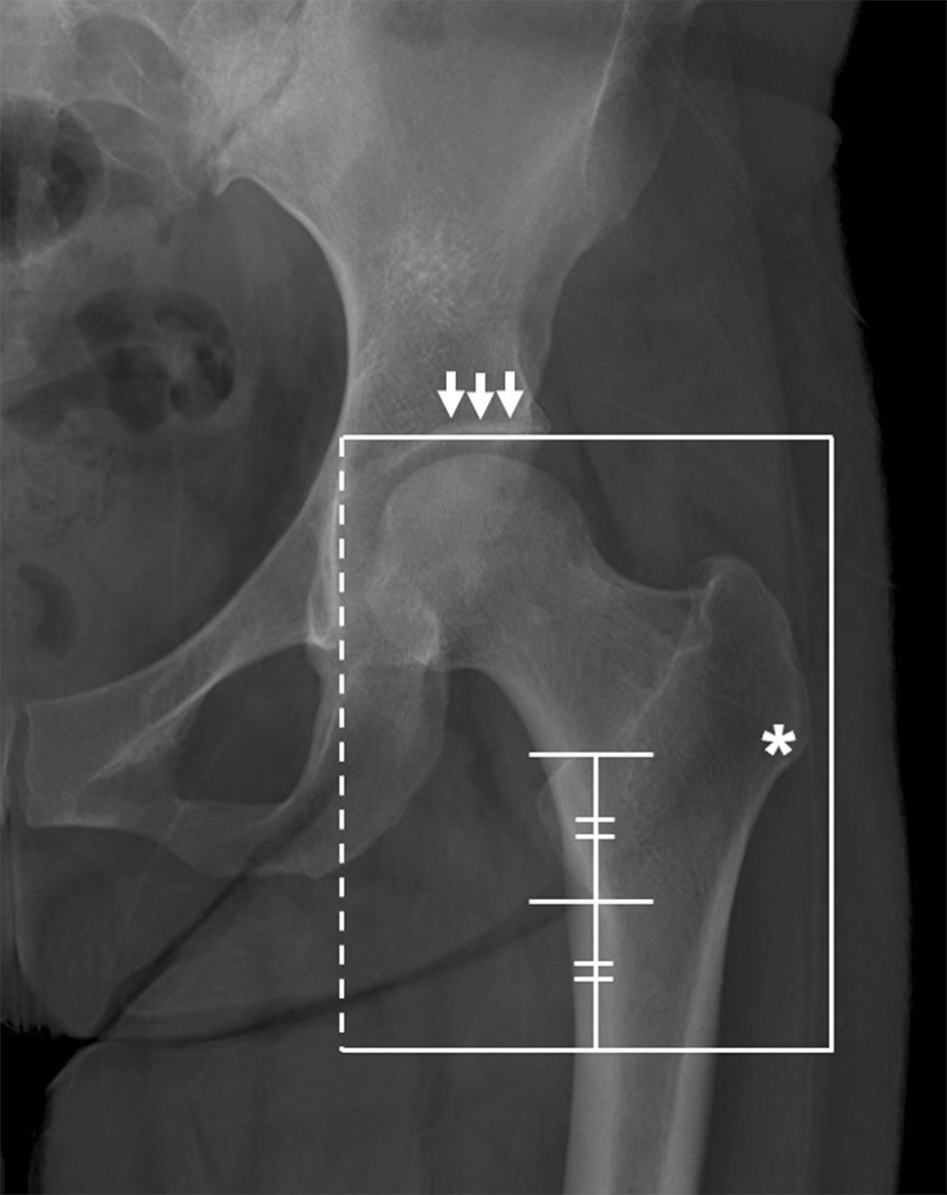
The region of interest that was cropped on simple hip radiograph. The upper border of the cropped image was the acetabular roof (arrows) and femoral head, and the lower border was located as far as the length of the lesser trochanter from the tip of it. The medial border (dotted line) was the crossing of the lateral margin of teardrop, and the lateral border of cropped image was positioned just lateral of the vastus ridge (asterisk).
